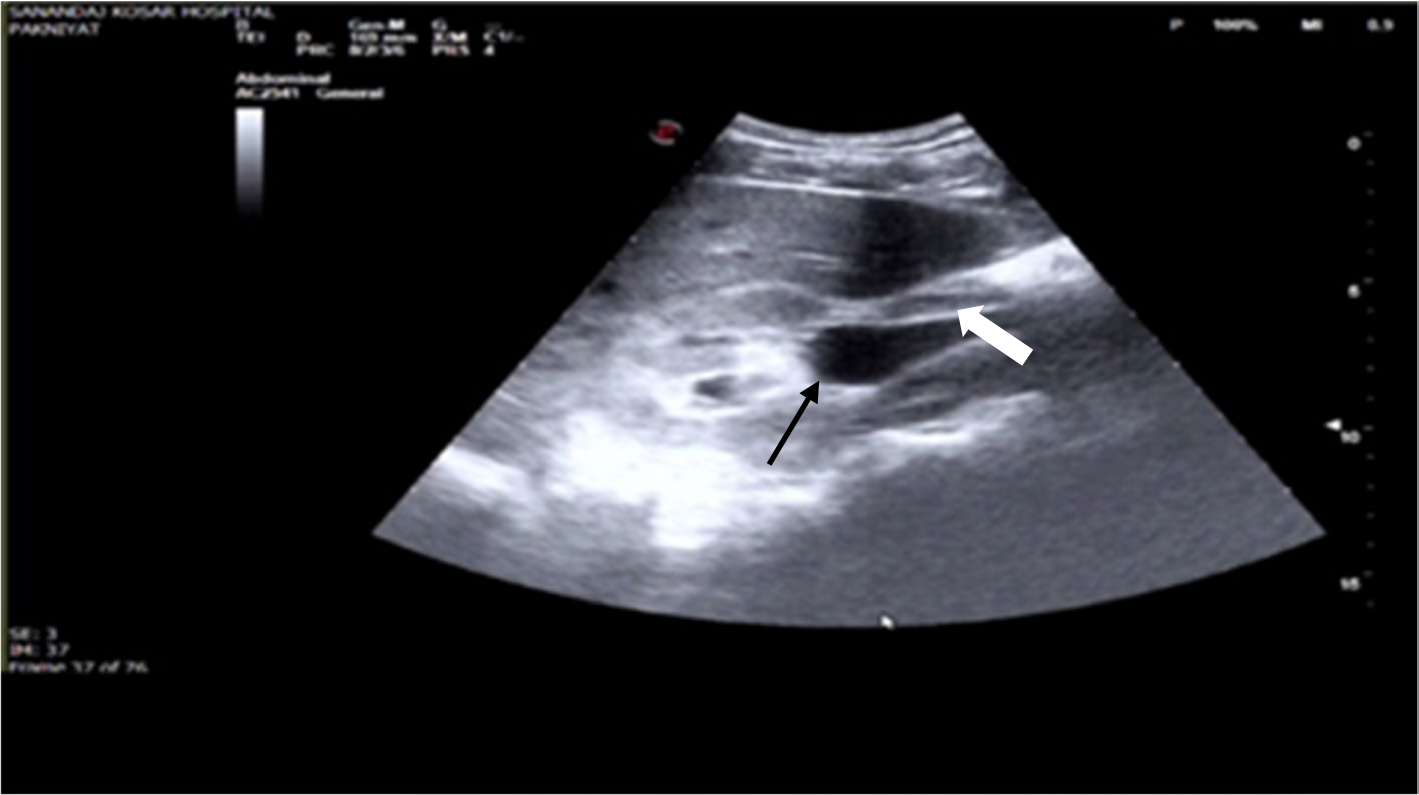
Ultrasound showing perinephric fluid and dilated ureter
